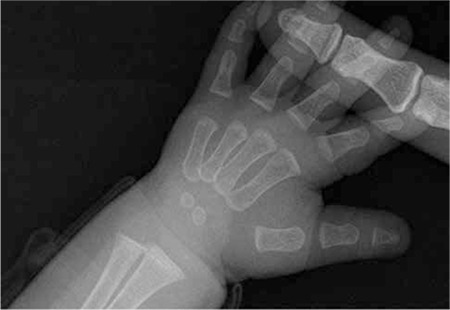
Case 2- improvement of metaphyseal irregularity and widening on wrist X-ray
